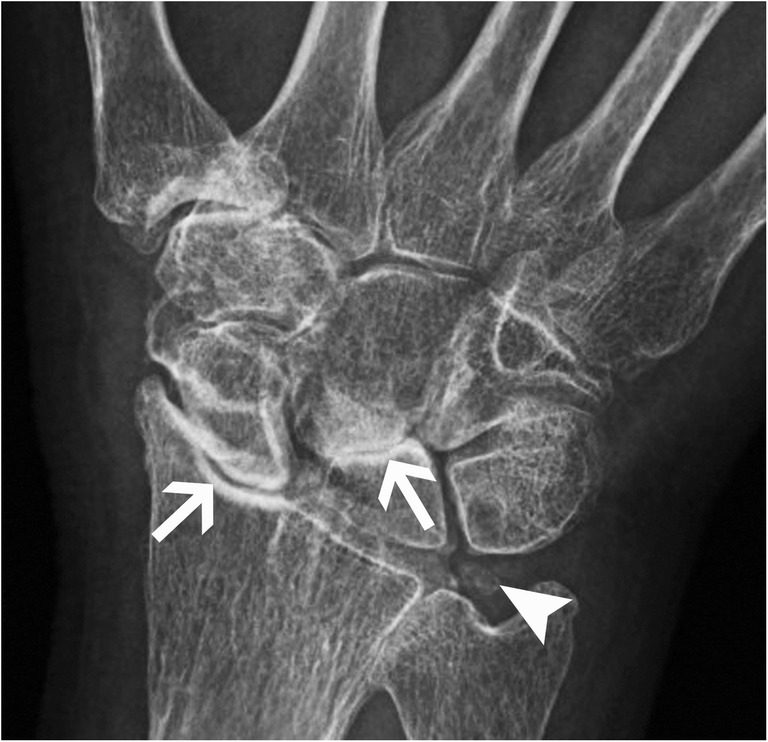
CPPD arthropathy of the wrist. Posteroanterior radiograph of the wrist shows joint space narrowing and dense subchondral sclerosis at the radioscaphoid and lunocapitate joints (arrows) associated with widening of the scapholunate space, consistent with a SLAC wrist. Note chondrocalcinosis at the ulnocarpal joint space within the triangular fibrocartilage (arrowhead)
